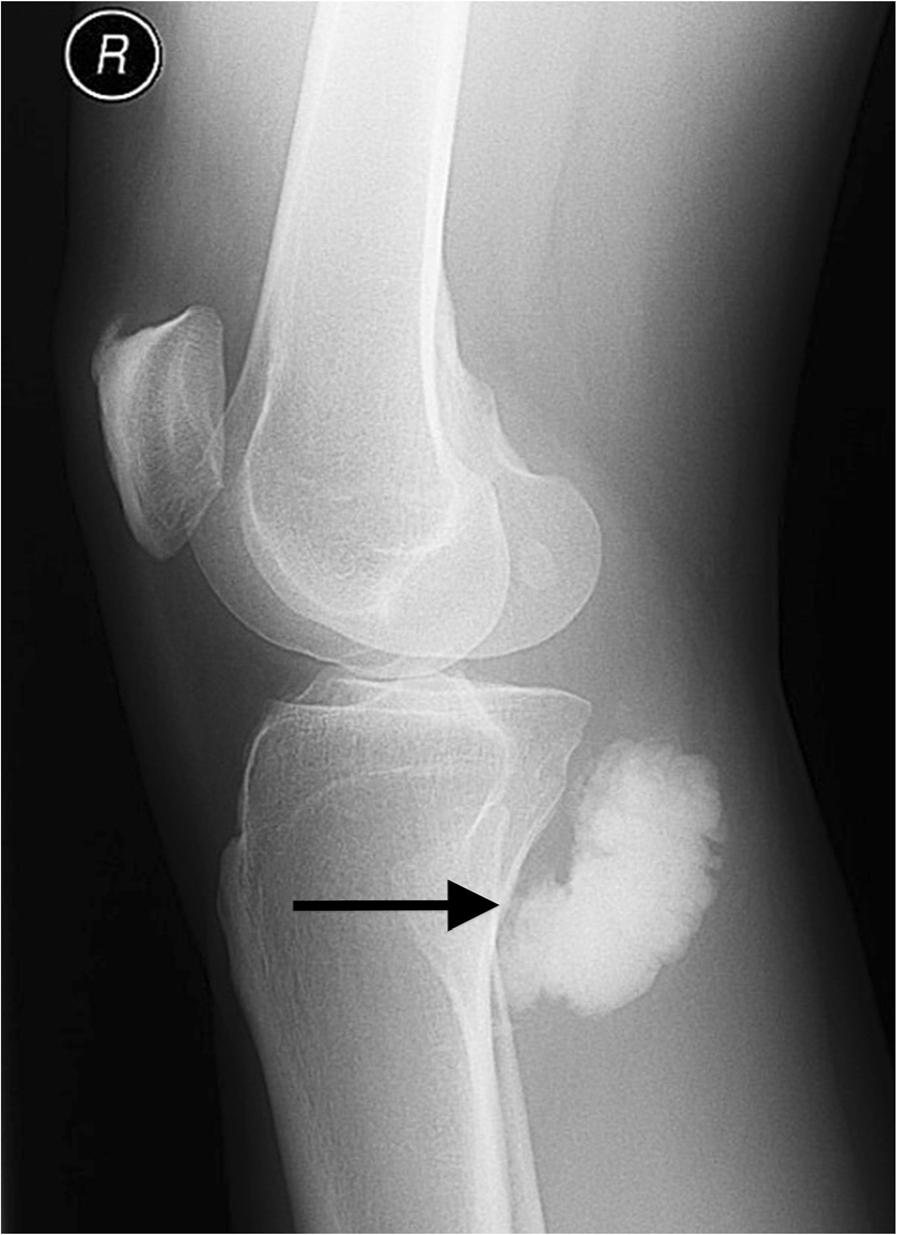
Lateral plain radiograph of the lesion.
